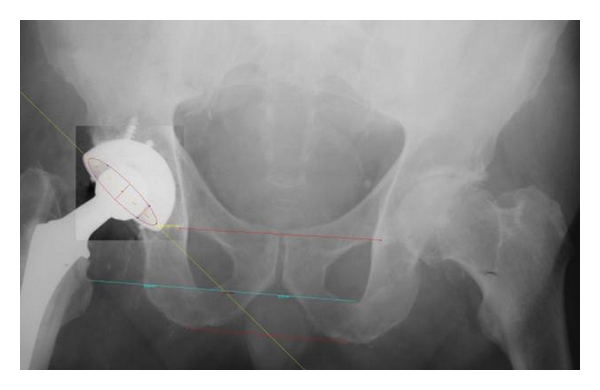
Digitalized representation of an AP radiograph centered over the pelvis as well as the determination of the inclination and anteversion using the software Imagika 1.50. To determine the anteversion, the ratio of the major and minor ellipses was used, which corresponds to the dark portion of the cup using the method of Ackland. The inclination of the cup was determined by measuring the angle between a line joining the ischial tuberosities or the teardrops and a line through the long axis of the ellipse.
